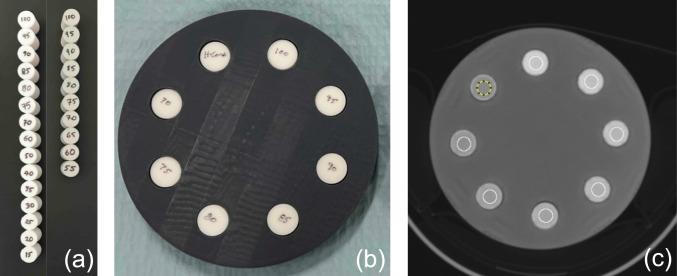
a Cylindrical white PLA (left) and StoneFil PLA (right) inserts with labelled infill densities. b PLA samples inserted into housing printed from standard grey PLA at 80% infill density. c CT slice of StoneFil samples with circular regions of interest for HU measurement
